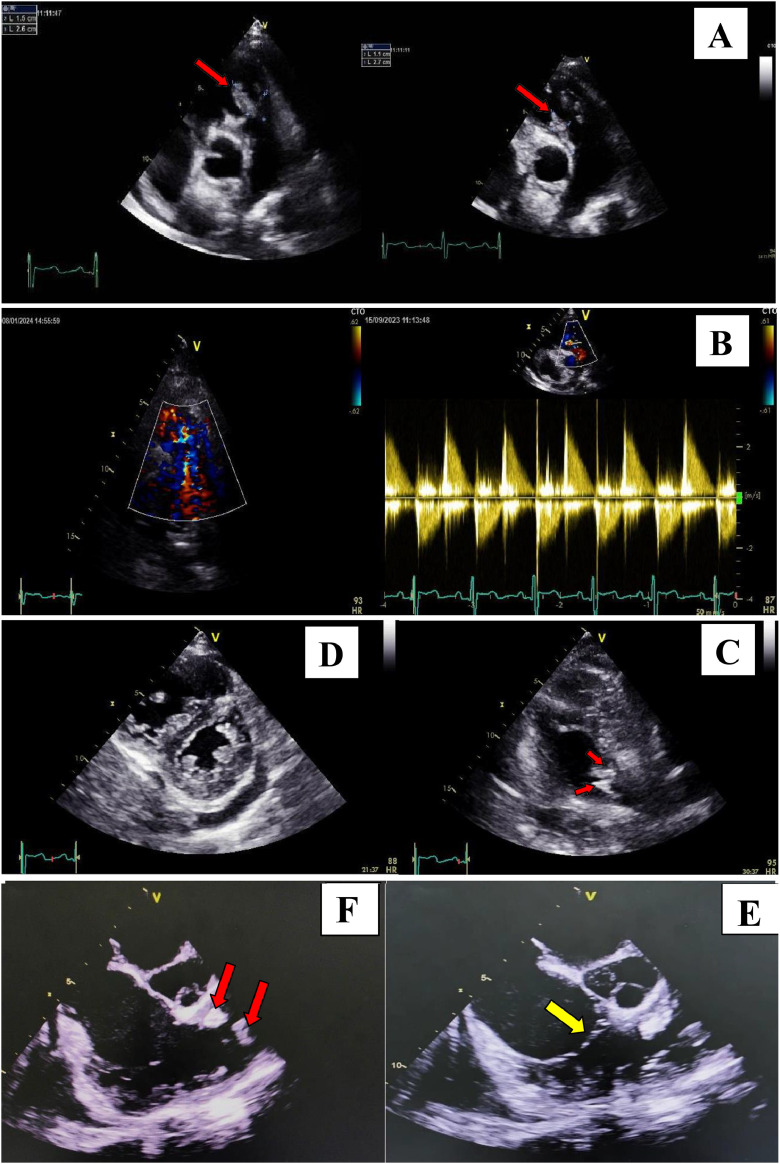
Transthoracic echocardiography (A, B, C, D) and transesophageal echocardiography (E, F) revealed 2 large vegetations (red arrows) attached to the pulmonary valve and severe pulmonary regurgitation. No vegetations were seen on the tricuspid valve (yellow arrow). The right ventricle was dilated with paradoxical septal motion with deteriorating right ventricle function (D).
